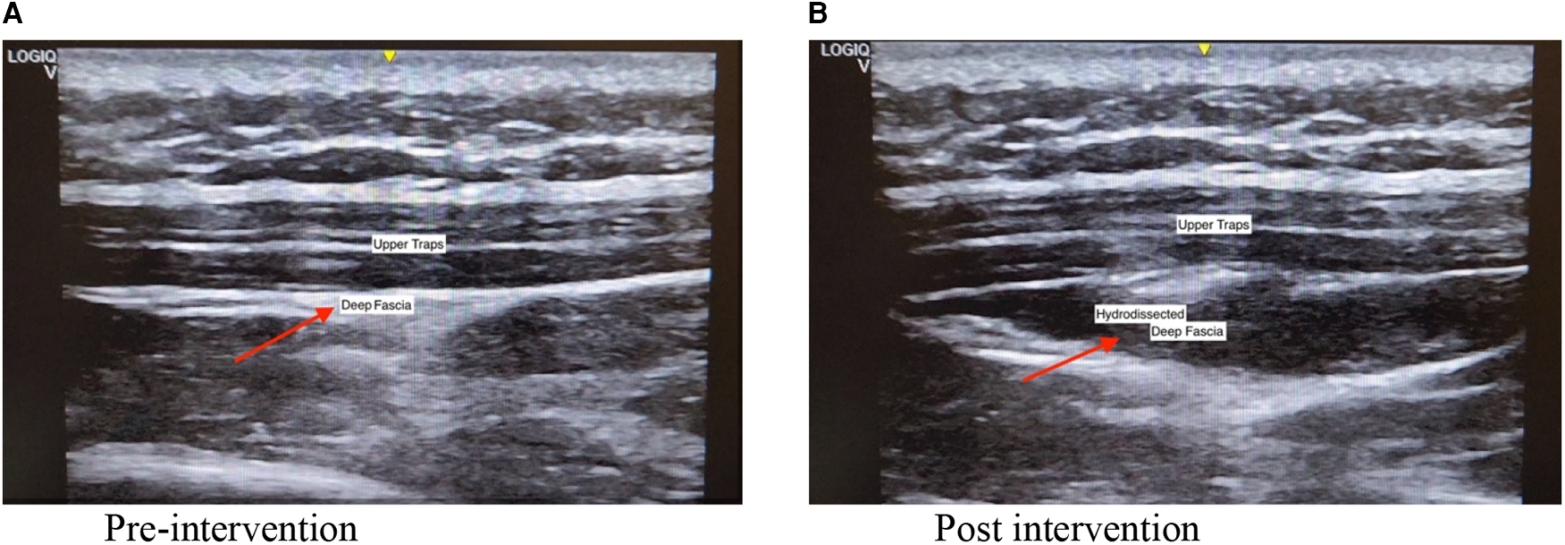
Ultrasound guided hydrodissection (A) pre-intervention (B) post intervention.
